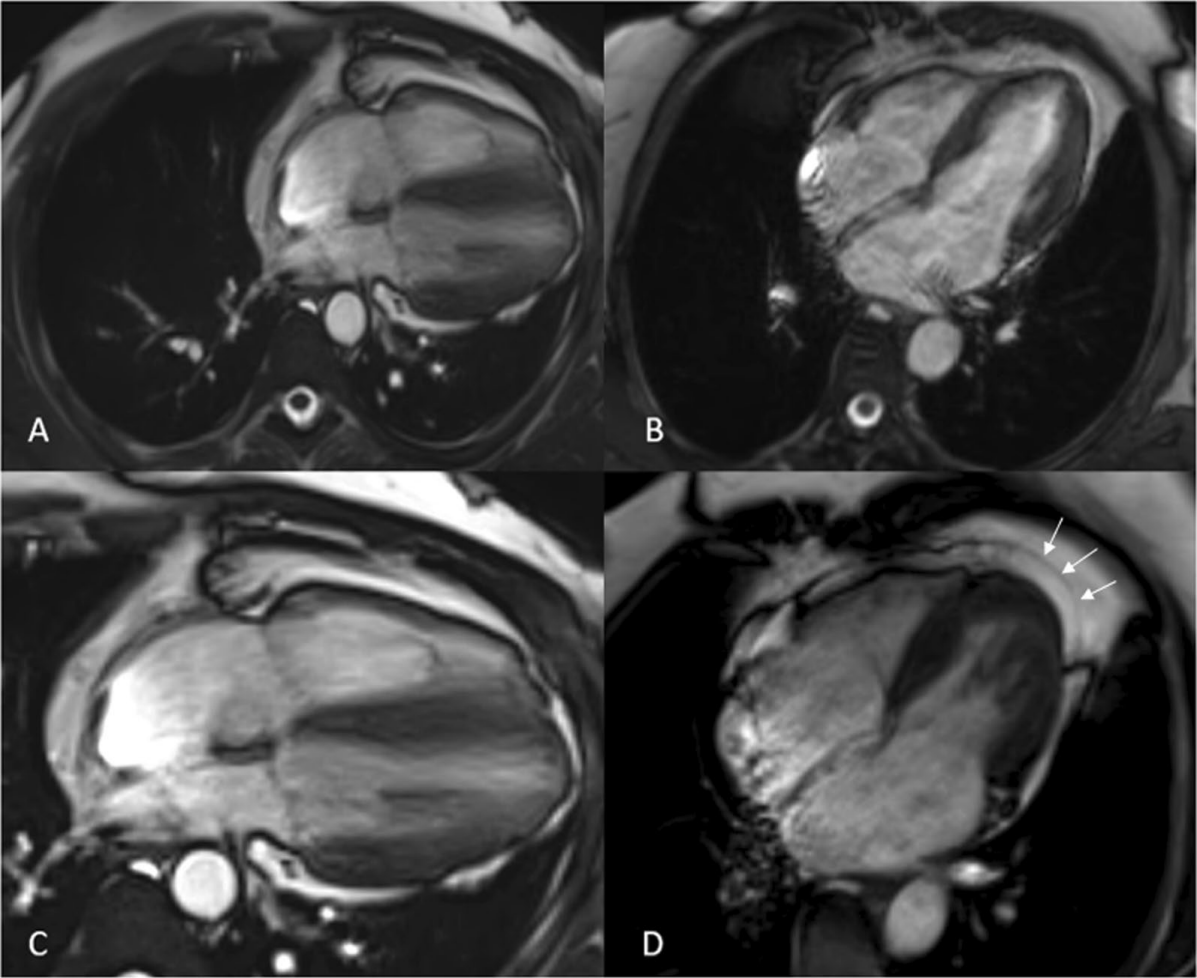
Images seen are comparisons of pericardial agenesis (left) with the normal cardiac MRI (right) for better understanding. Image A CINE image of four-chamber view showing excessive levorotation of the heart in comparison to the image B which is of normal patient showing normal location of the heart. Image C CINE image of four-chamber view reveals non-visualization of pericardium in the apical and posterior cardiac margins, which is clearly seen in the image D revealing normal cardiac MRI marked with white arrows
